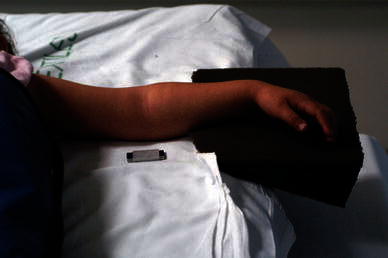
Internal oblique radiograph positioning: arm extended, forearm pronated, anterior surface of elbow at 45 degrees
